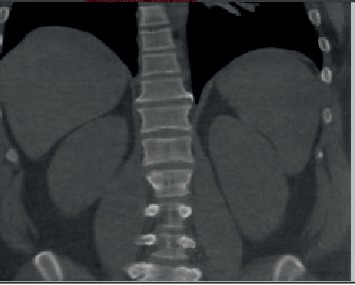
CT enterography revealing jug-like syndesmophytes at T12 and L1, suggestive of spondyloarthropathy.
